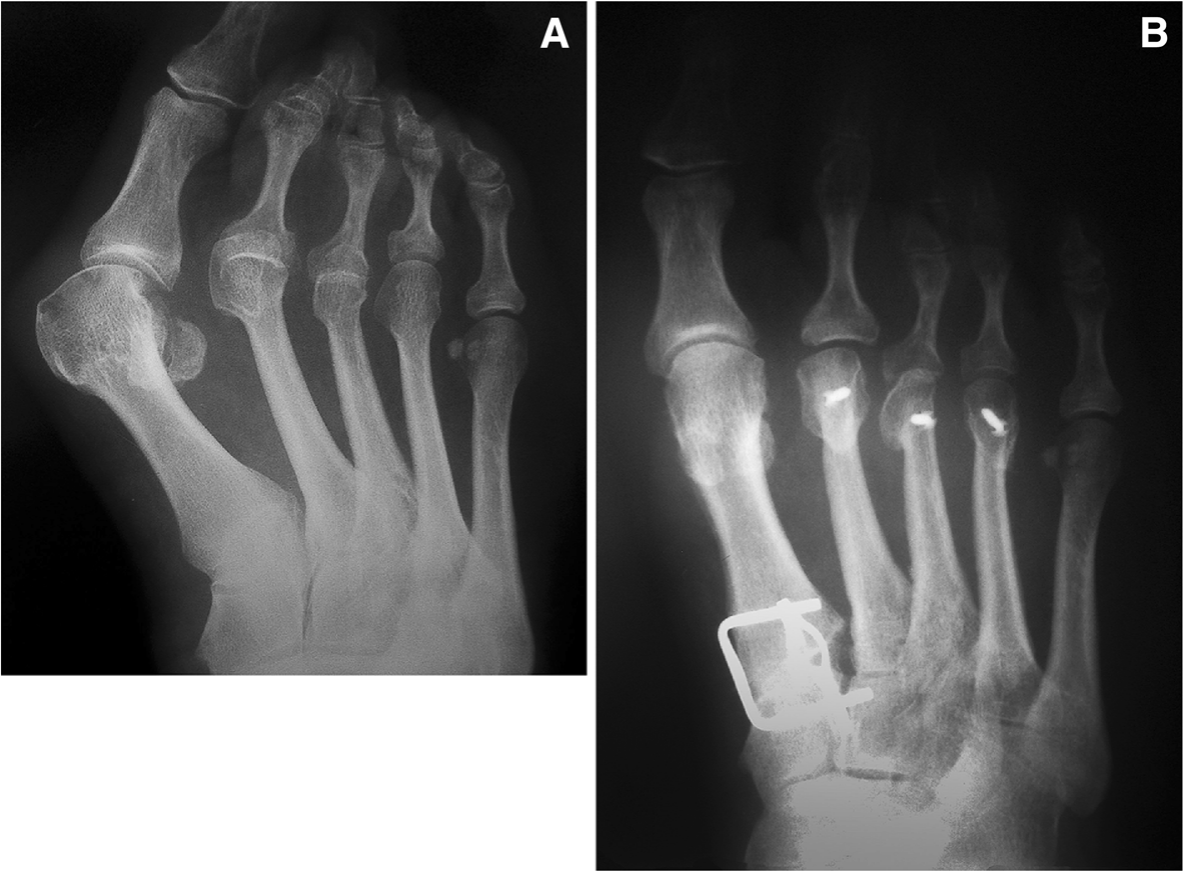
A Preoperative X-ray. Preoperative anteroposterior X-ray of right foot shows subluxation of the first metatarsophalangeal joint and dislocation at the second and third metatarsophalangeal joints. B. Postoperative X-ray. Postoperative anteroposterior X-ray of right foot shows the arthrodesis of the medial cuneometatarsal joint fixed by two memory staples and Weil osteotomy of the second to fourth metatarsal bones. Subluxation of the first metatarsophalangeal joint was resolved by the lateral release of the joint.
